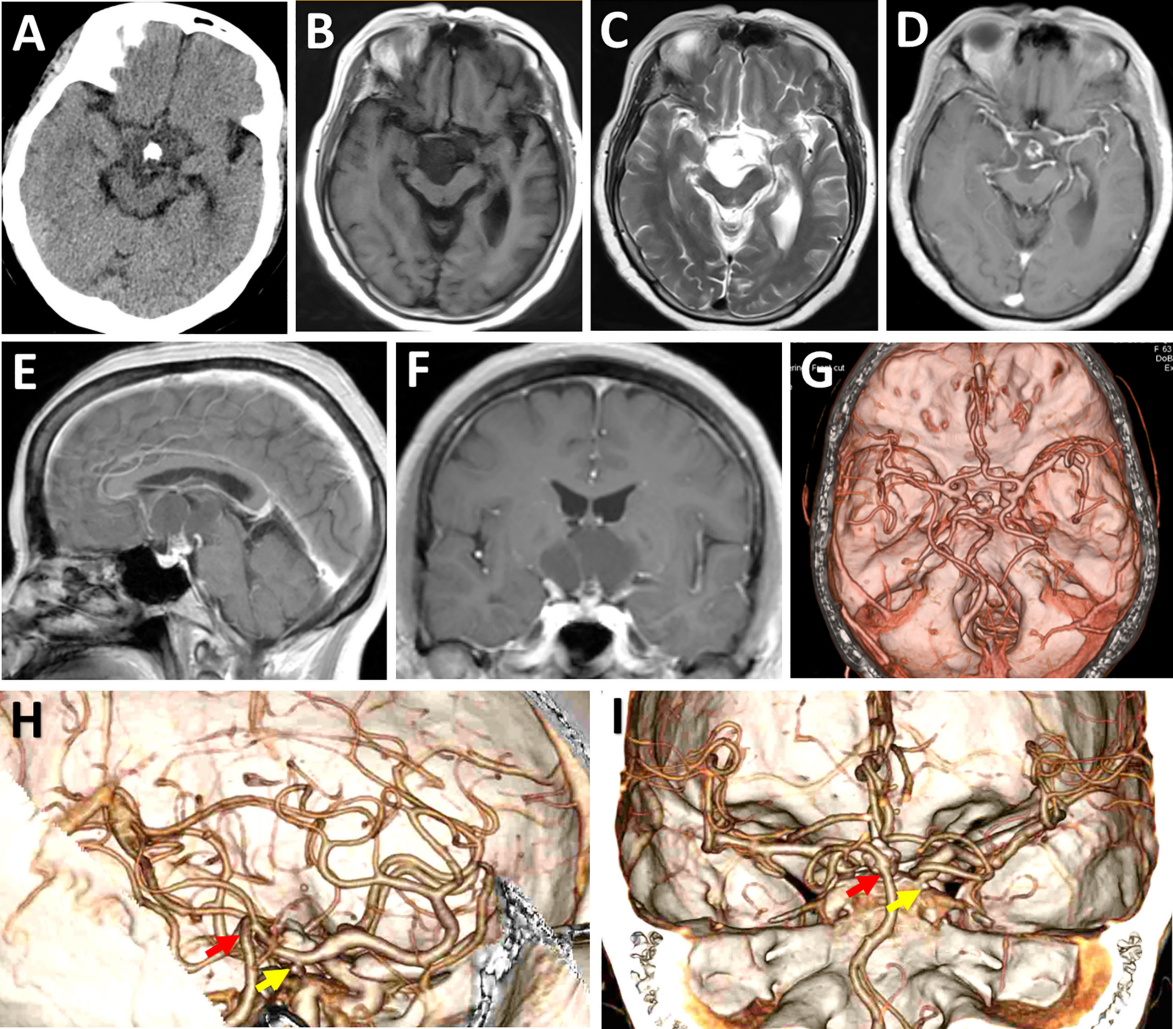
Radiological evaluation of the suprasellar lesion preoperatively. (A-F) Preoperative CT and MRI scans showing a typical retroinfundibular CP. This lesion manifested as a hypodense irregular mass with calcification located in the suprasellar lesion (A), which was hypo-intense on T1WI (B) and hyper-intense on T2WI (C). Post-contrast (gadolinium-enhanced) axial (D), sagittal (E), and coronal (F) MRI showed a thick, irregular enhancement of the solid part and a slight rim enhancement of the cystic component. (G-I) Preoperative axial (G), sagittal (H), and coronal (I) CT angiography images showed the relative location of the tumor to the Willis’ circle. Note that in this patient the basilar apex was at a higher position surpassing the posterior clinoid process. The yellow arrows denote posterior clinoid process, while the red arrows depict the basilar apex.
